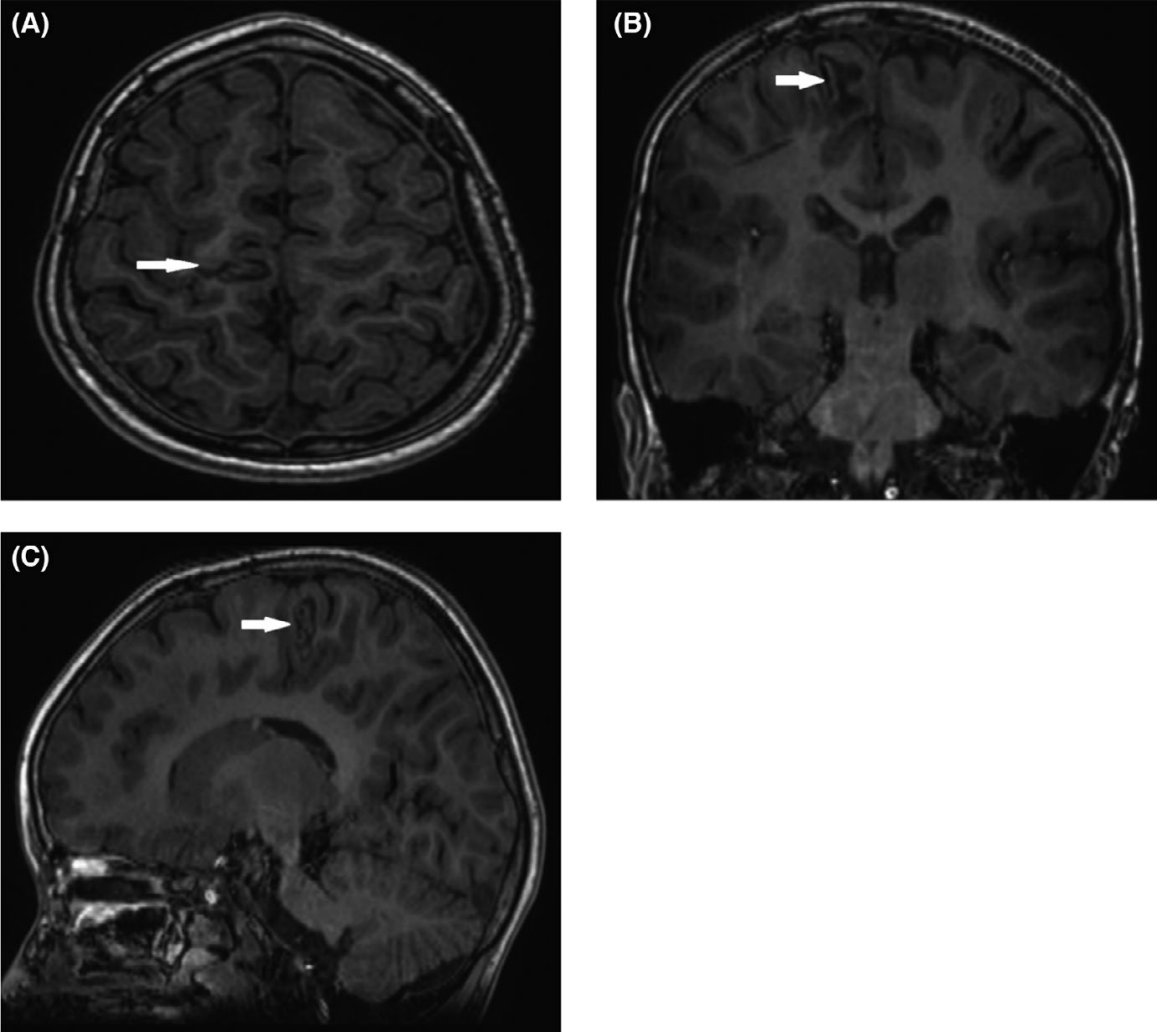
Postoperative MR images were obtained 6 months after the second stage of high‐density RF‐TC in patient No. 8. The expanding coagulated lesion (white arrow) at the nodule of the right paracentral lobule was shown (A, axial image; B, coronal; C, sagittal)
